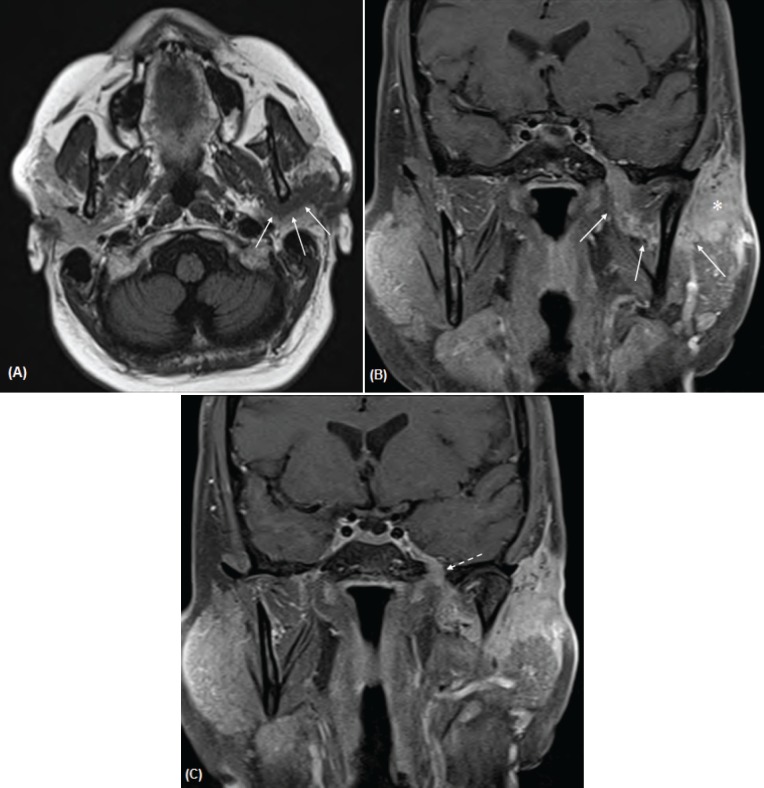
Axial T1-weighted image (A) and post-contrast coronal T1-weighted images with fat saturation (B, C) demonstrates a curvilinear band of enhancing tumour (arrows in A, B) that extends from the left parotid mass (asterisk) and tracks behind the mandibular ramus and joins with the V3 nerve in the masticator space. There is contiguous extension along V3 superiorly through a widened foramen ovale (dashed arrow in C) and asymmetric thickening and enhancement of the left cavernous sinus.
